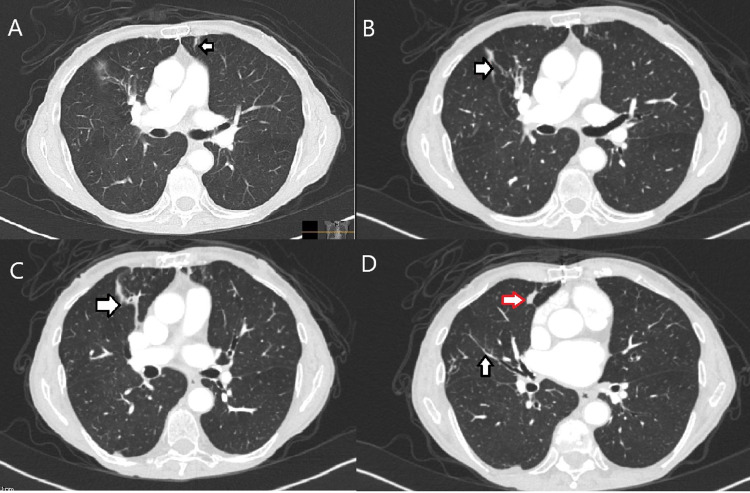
CT restaging scan (A) Left upper lobe nodule. (B and C) Parenchymal opacities with tree/budding opacities demonstrating underlying bronchiectasis. (D). Tree/bud opacities (black arrows) with distal mucus plugging (red arrow).
